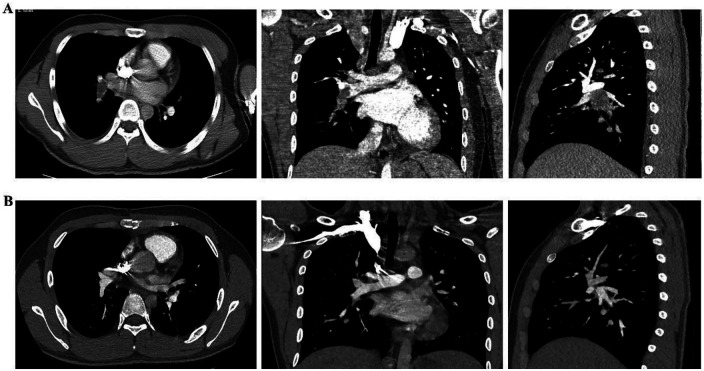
Pulmonary artery CTA showing the preoperative and postoperative conditions of pulmonary artery thrombosis. (A) Preoperative pulmonary artery CTA; (B) postoperative pulmonary artery CTA.
